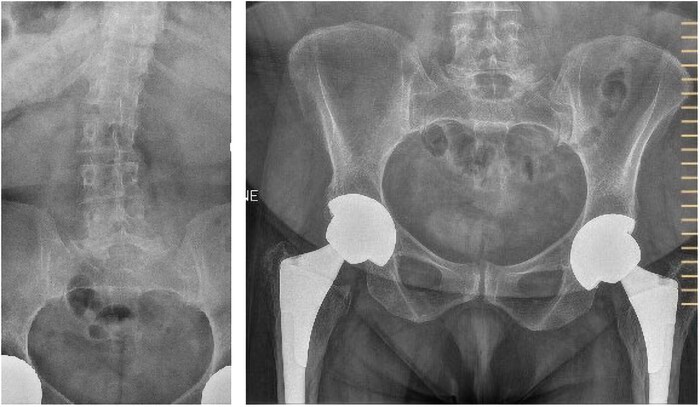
Skeletal findings age 52. Lumbar x-ray with diffuse osteopenia and mild scoliosis. Pelvis shows bilateral hip arthroplasty and diffuse osteopenia.
